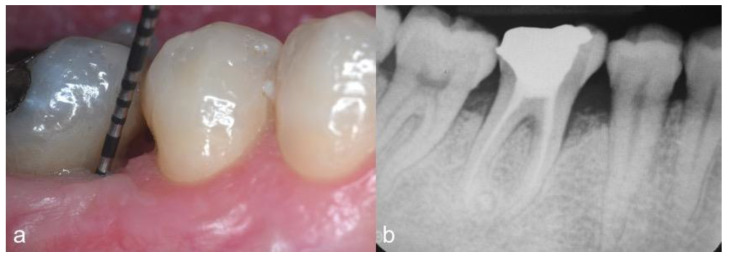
(a) 12-month follow-up clinical view showing significant PD reduction (residual PD of 3 mm); (b) 12-month follow-up periapical radiographic view showing filling of the infrabony component of the defect.
